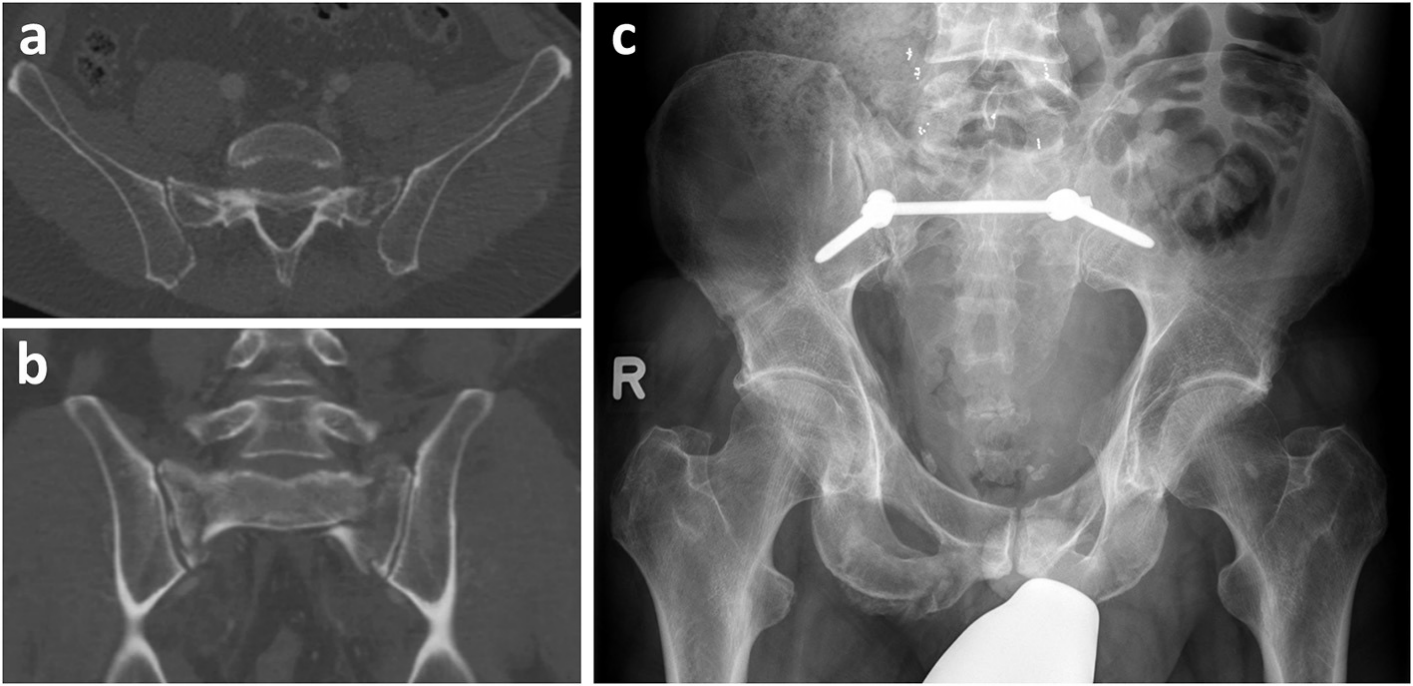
TIFI. Male patient (53 years old) after fall from greater height. Pelvic injury was classified (a: CT scan coronal, b: transversal) as AO C2.1b2c3. After initial stabilization with external fixator, definitie surgery was performed with transiliac fixation (c).
